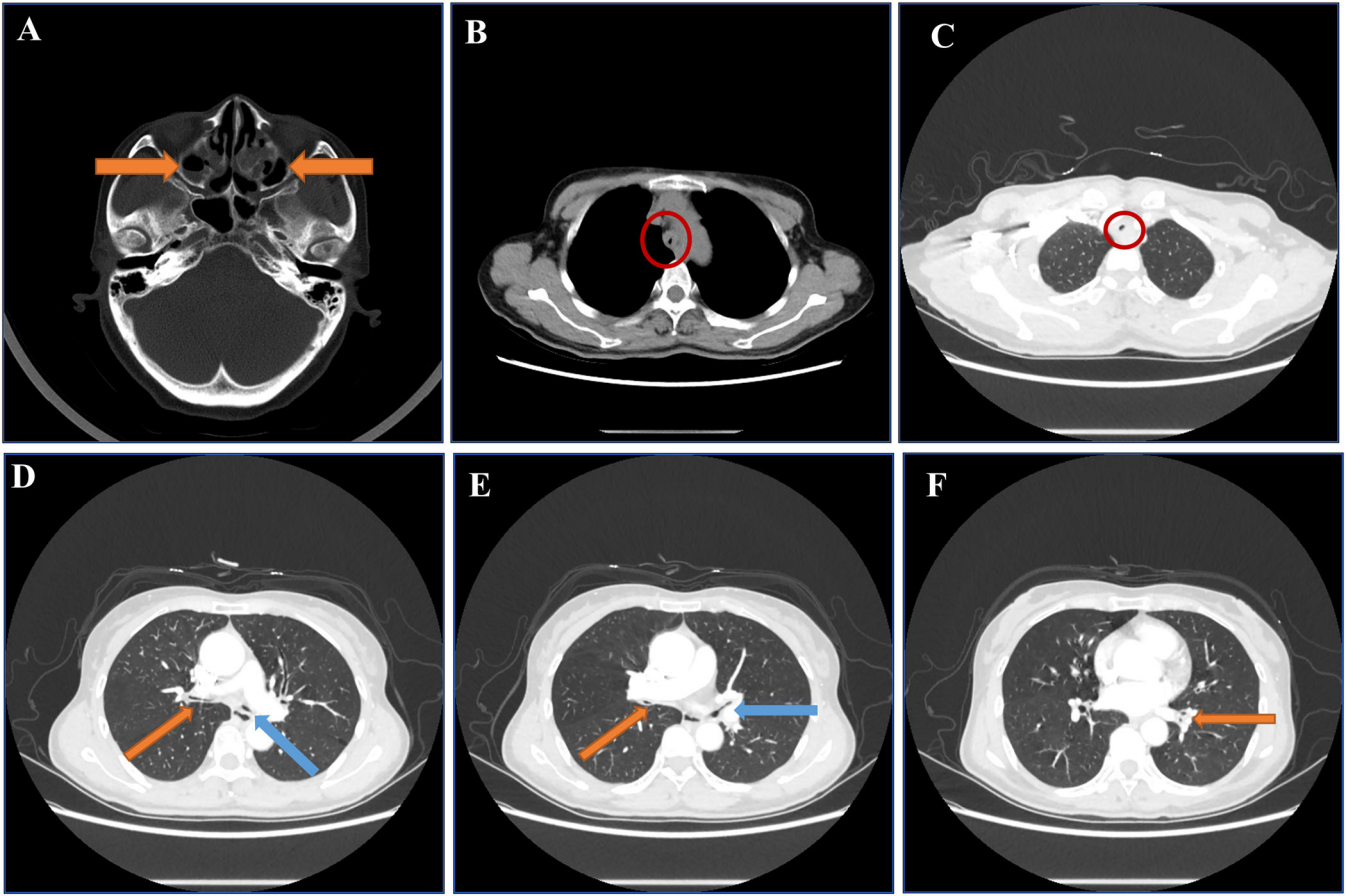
Sinus and chest CT scan. (A) Axial sinus CT scan demonstrated thickened mucous membranes in the bilateral maxillary and ethmoid sinuses (orange arrows), indicating sinus involvement. (B and C) Axial chest CT (Brilliance iCT; Philips Healthcare, Cleveland, Ohio, USA) scans revealed tracheal wall thickening (~5 mm) with tracheal lumen narrowing, with the narrowest portion measuring ~4–5 mm (red circles). (D) Axial chest CT scan indicated the narrowest points of the right (1–2 mm, orange arrow) and left main bronchi (1–2 mm, blue arrow). (E) Axial chest CT scan depicted narrowing of the right middle bronchus to about 1 mm (orange arrow) and the left upper lobe bronchus to ~2 mm (blue arrow). (F) Axial chest CT scan showed the left lower lobe bronchus narrowed to about 2 mm (orange arrow).
